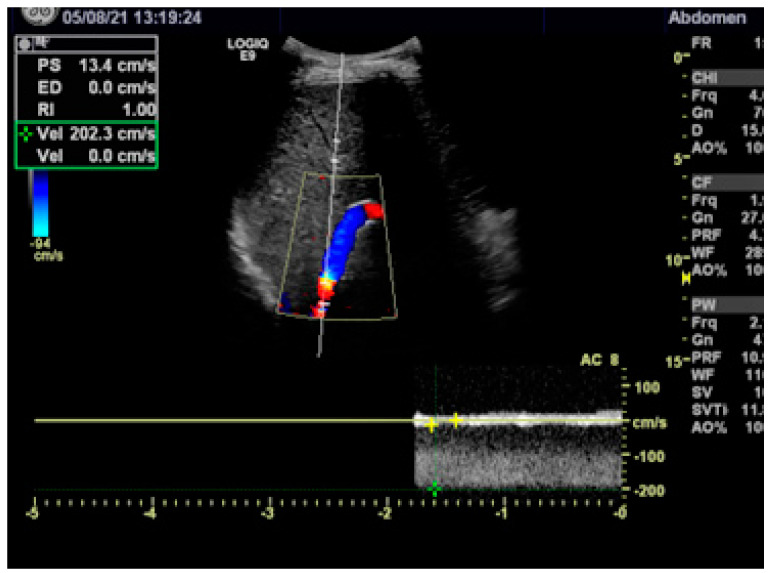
Duplex sonography showing stenosis in the proximal stent with a turbulent flow and a maximum flow velocity of 200 cm/s (2 m/s). According to the Bernouilli equation, the maximum flow velocity corresponds to a pressure gradient across the stenosis of 16 mmHg.
